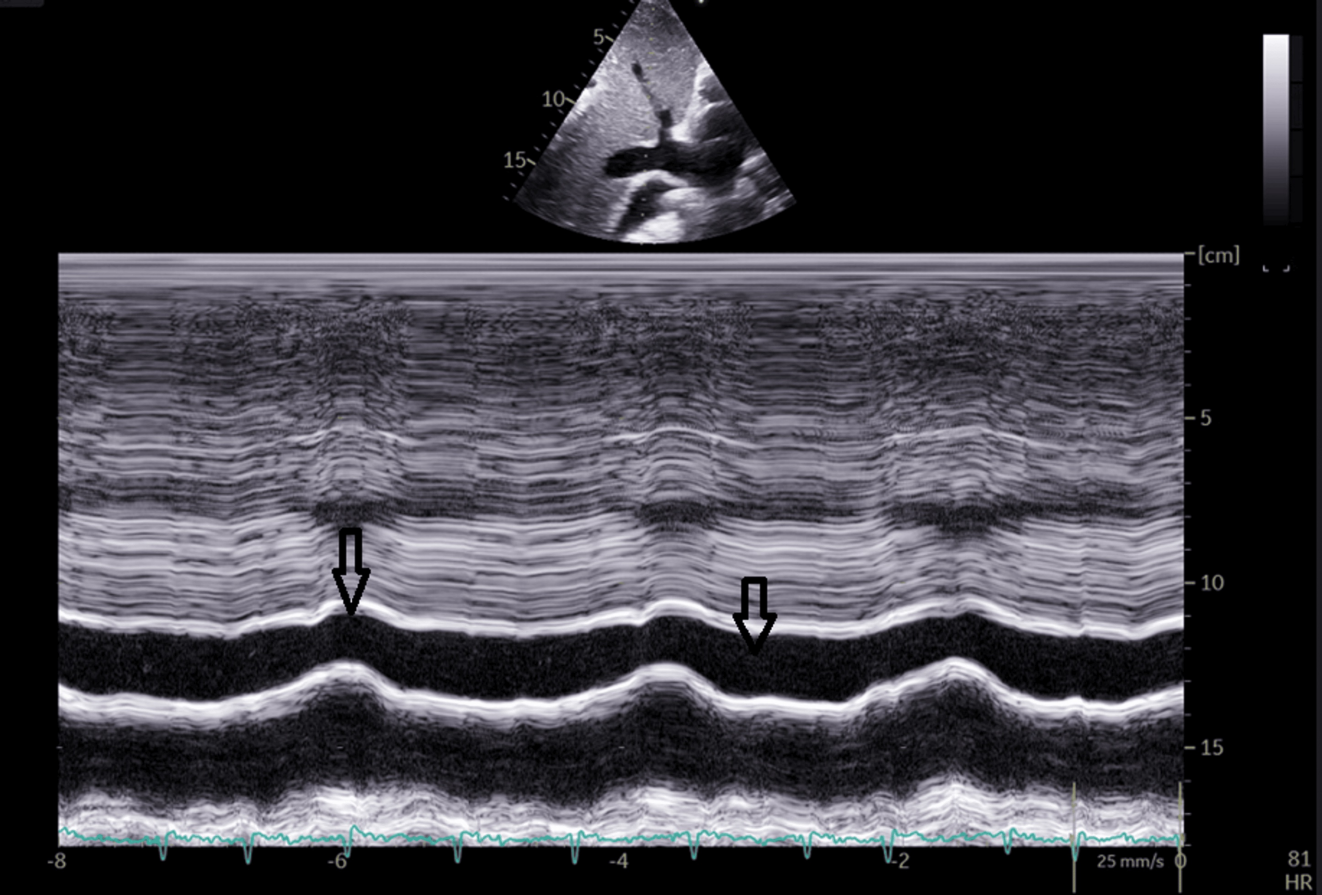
Echocardiography In the subcostal view, in M-mode, the enlarged inferior vena cava without inspiratory collapse is highlighted
